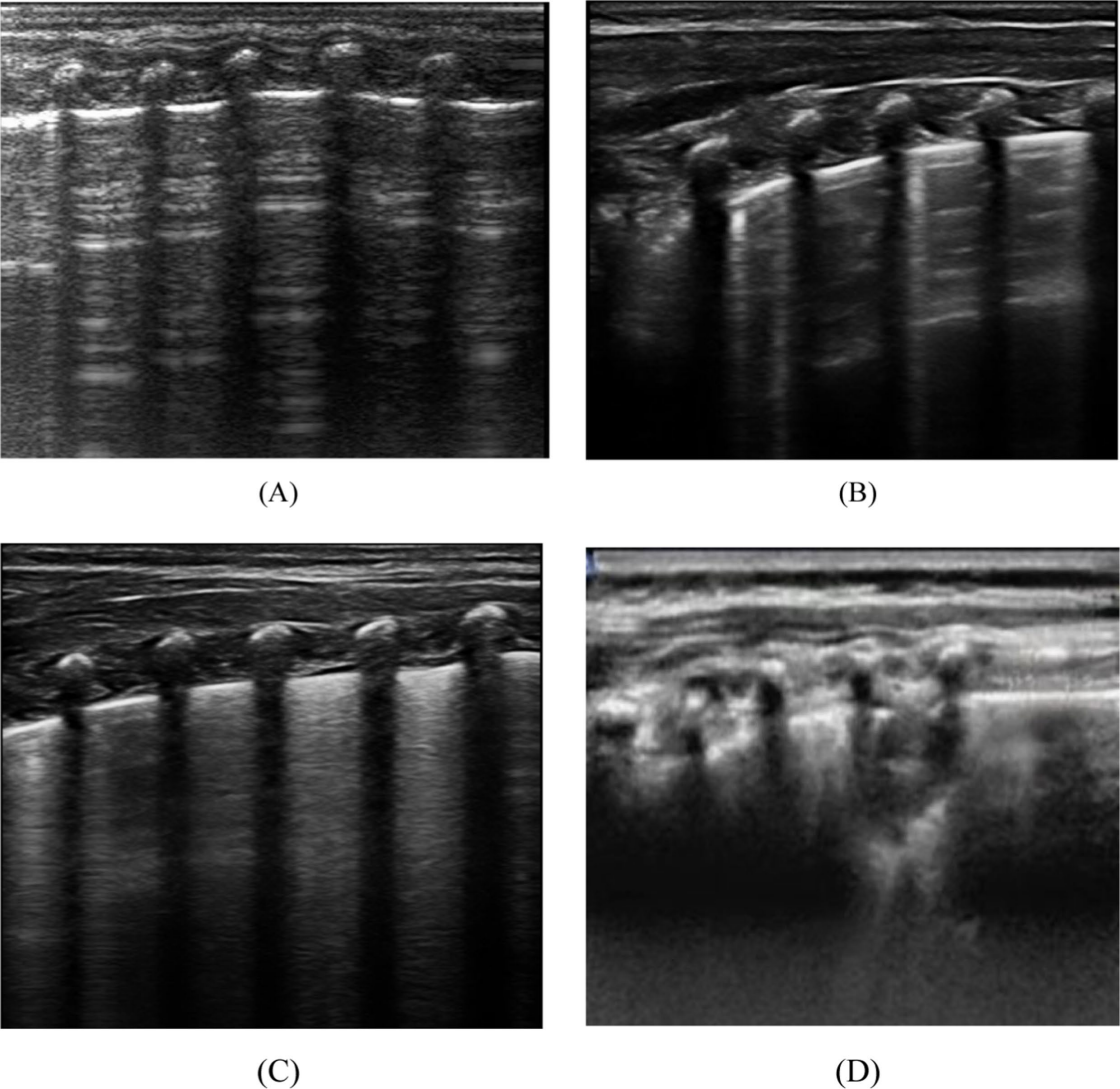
Depicts the four distinct lung ultrasound scoring patterns. (A) A score of 0 is assigned when only A lines are visible or fewer than 3 B lines. A vertical arrow indicates A-lines. (B) A score of 1 is given when there are 3 or more well-spaced B-lines, shown with horizontal arrows. (C) Score 2 was line B with many fusions, with or without subpleural consolidation. (D) 3 points are defined as “hepatoid” lung tissue or accompanied by an air bronchogram, represented by an oval.
